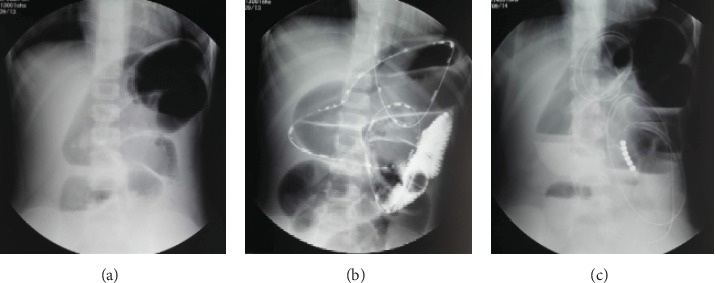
Presentative abdominal X-ray plain films of the TIT procedure. (a) Before intubation, the proximal intestinal canal dilated obviously according to the plain films. (b) The patient was treated with the TIT procedure, and the intubation depth was 135 cm. (c) 24 hours after intubation, the dilatation of intestine was relieved.
